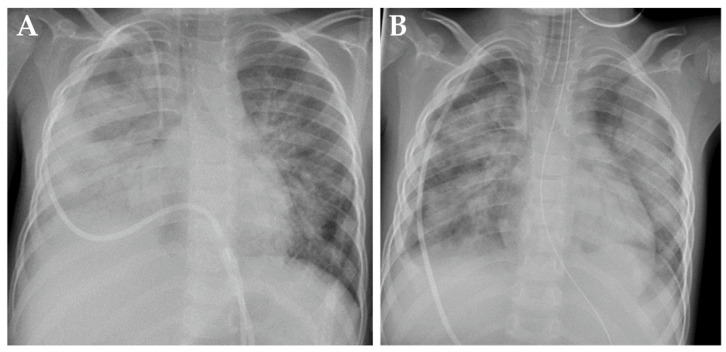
Chest X-rays from patient 1 demonstrating signs of busulfan-induced lung injury on day +62 (A) and day +74 (B) after high-dose chemotherapy.
